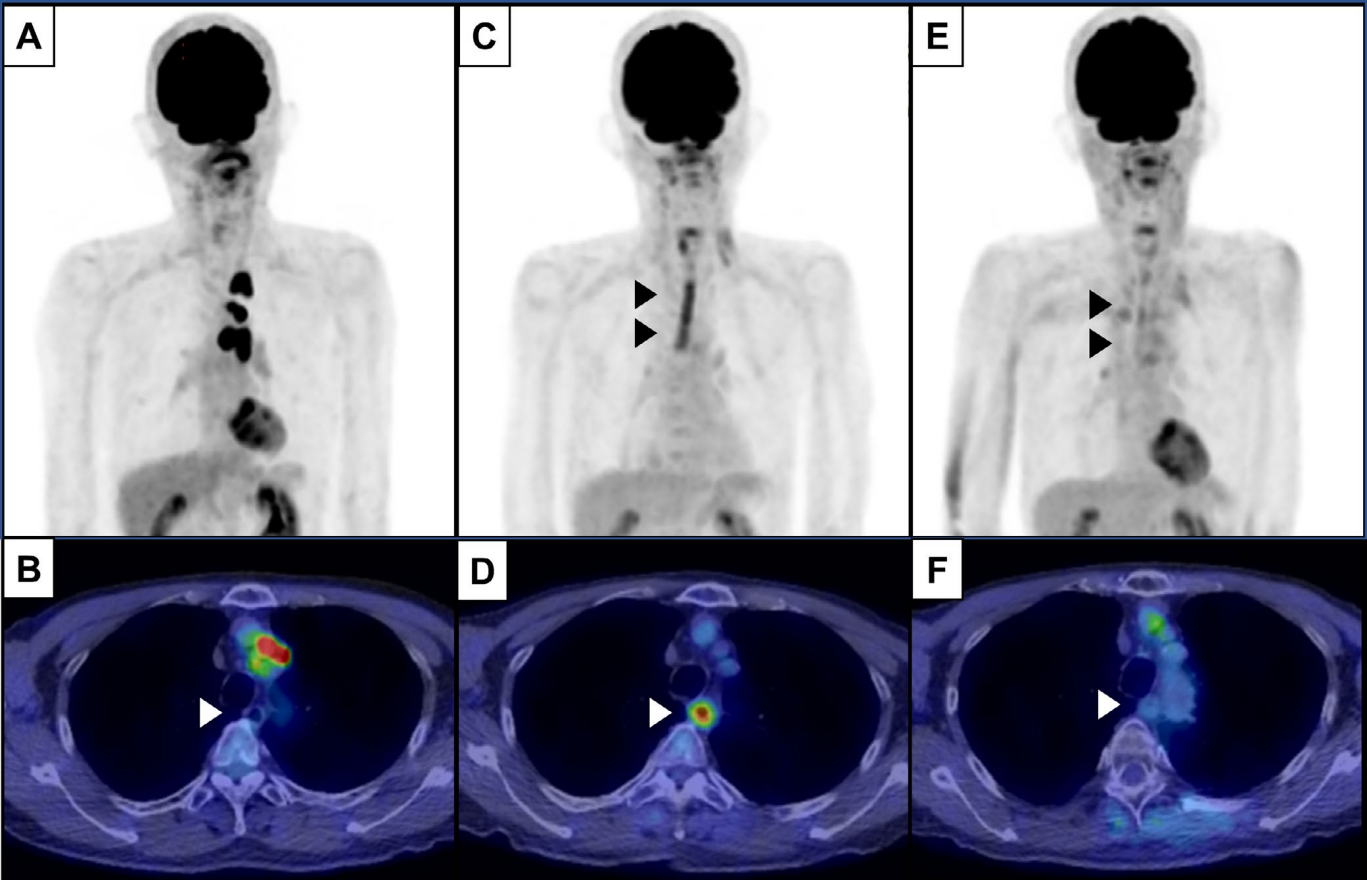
(A, B) PET imaging shows increased [18F]‐FDG uptake in the tumor located in the left upper lobe and the ipsilateral mediastinal lymph nodes. (C, D) One month after completion of definitive chemoradiotherapy, PET revealed abnormal FDG uptake (SUVmax = 5.5) along the esophagus in a tube‐like pattern (arrowheads). (E, F) Seven months after treatment, the abnormal esophageal FDG uptake had completely resolved (arrowheads).
